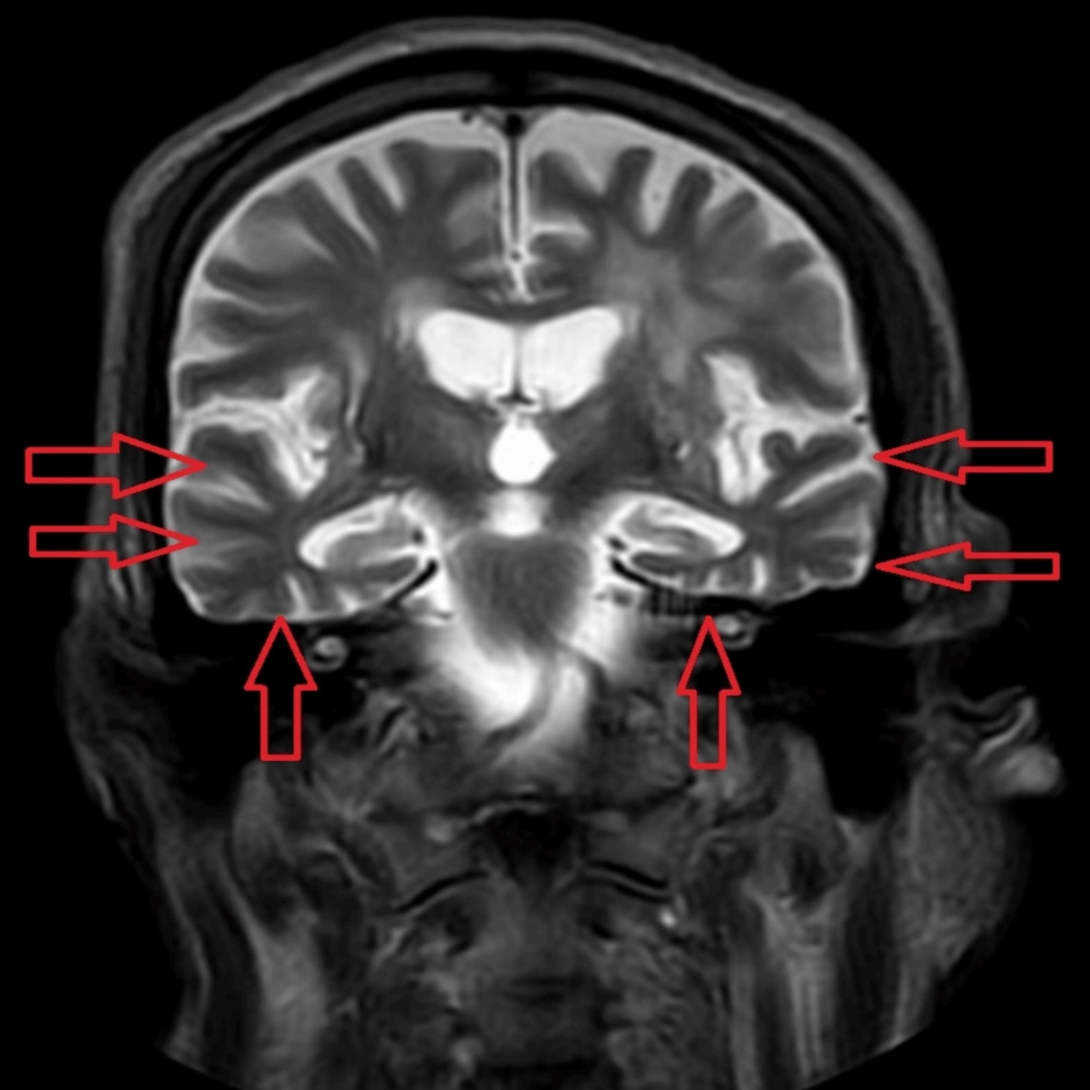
MRI in T2-weighted coronal section showing age-related atrophic changes in the bilateral frontotemporal lobe with thinning of the bilateral superior, middle, and inferior temporal gyri (red arrows)
